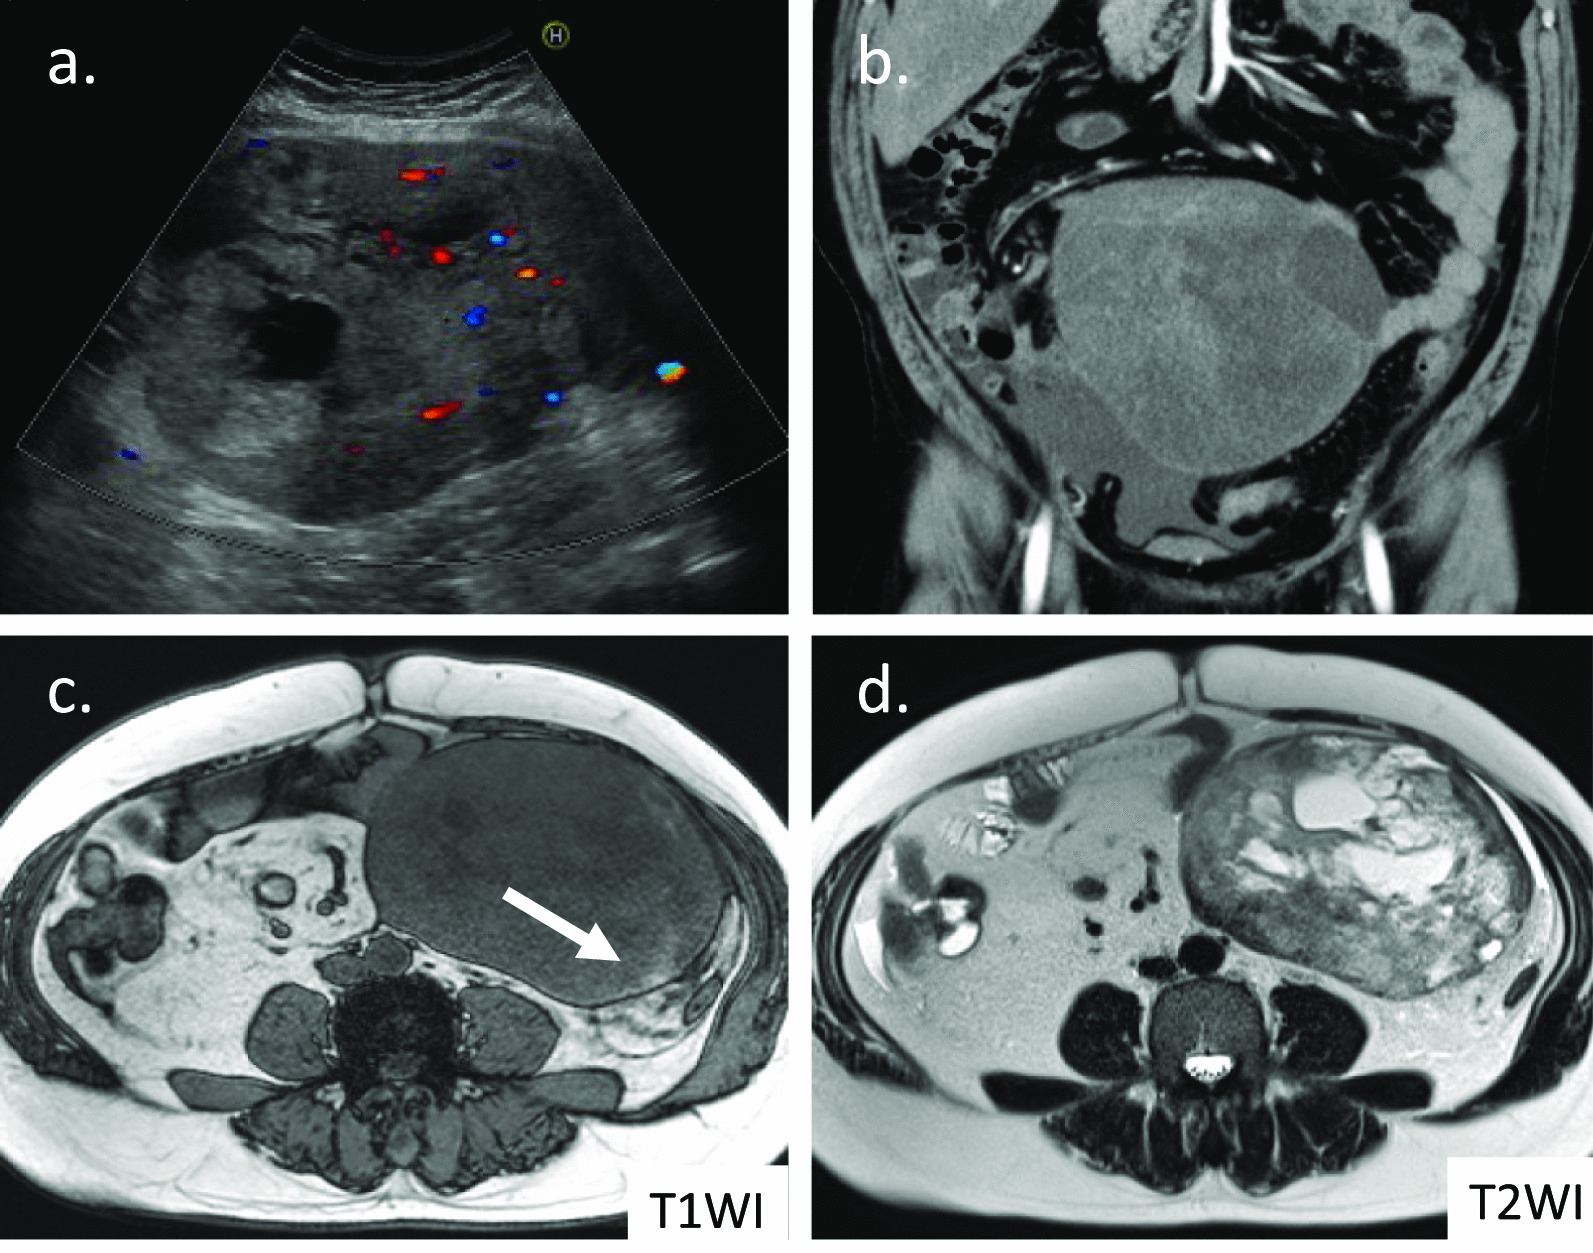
Diagnostic findings in Case 1. a Abdominal ultrasonography displaying a large solid mass with cystic degeneration, and an irregular echoic pattern with vascular signals inside. b Abdominal contrast-enhanced computed tomography displaying a large, well-defined circular tumor with a heterogeneous interior, which appears to be partially continuous with the small intestine. c Magnetic resonance imaging displaying some high-intensity signals (white arrow) on T1-weighted image. d Cyst components are scattered on T2-weighted image
